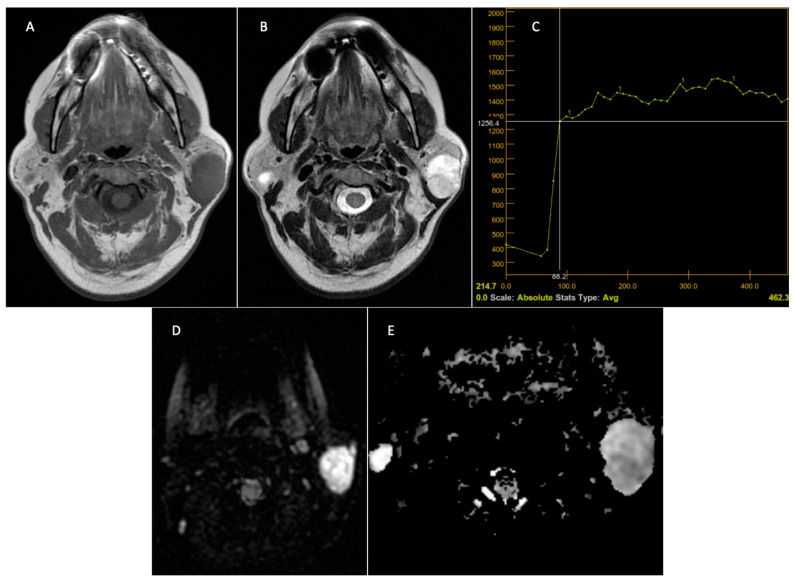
Pleomorphic adenoma—left parotid gland. Preoperative MRI. T1 sequence with a hypointense signal (A). T2 sequence with a discrete inhomogeneous hyperintense signal (B). DCE-MRI—slow and progressive contrast uptake with late washout—TIC curve type A, typical appearance of pleomorphic adenoma (C). The DWI—MRI sequences (D) demonstrate T2 appearance, “shine through”, with a hypersignal on the DWI sequence and ADC, with the ADC map (E) having a value of 1.6 × 10^–3^ mm^2^/s.
